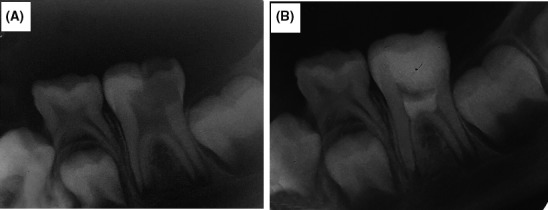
(A) preoperative radiograph showing the left mandibular molar with open apex and deep carious lesions, (B) postoperative radiograph after MTA placement canal orifices and the floor of pulp chamber
